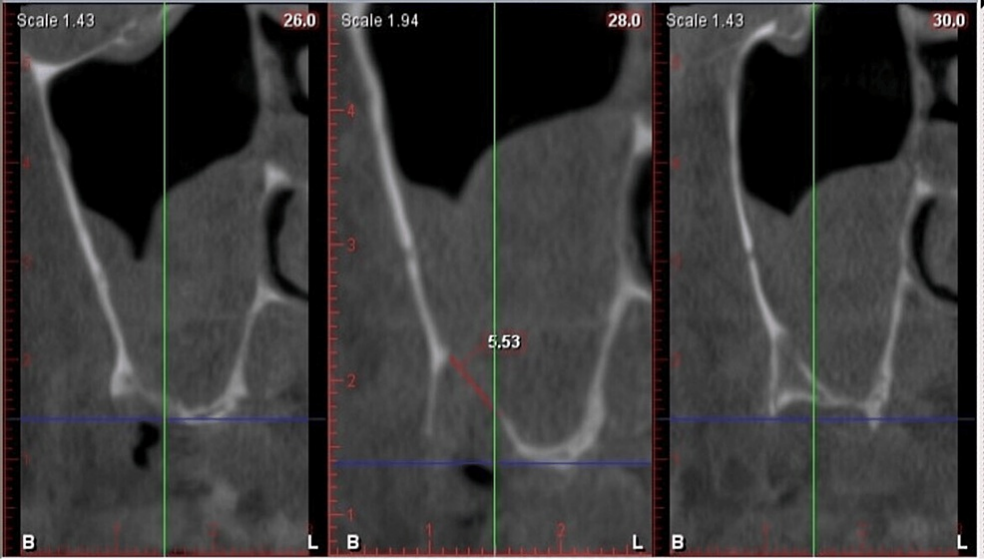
Cone-beam computed tomography after the surgery: paraxial slices (day 5) (Case 1)
